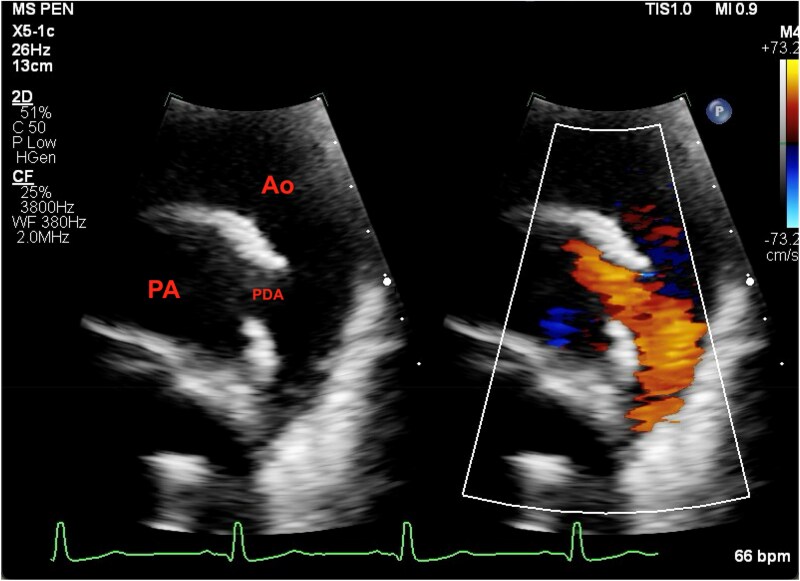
Echocardiogram after the patient received 4 months of pulmonary vasodilator therapies. Flow through the PDA demonstrates low-velocity left-to-right shunt.
